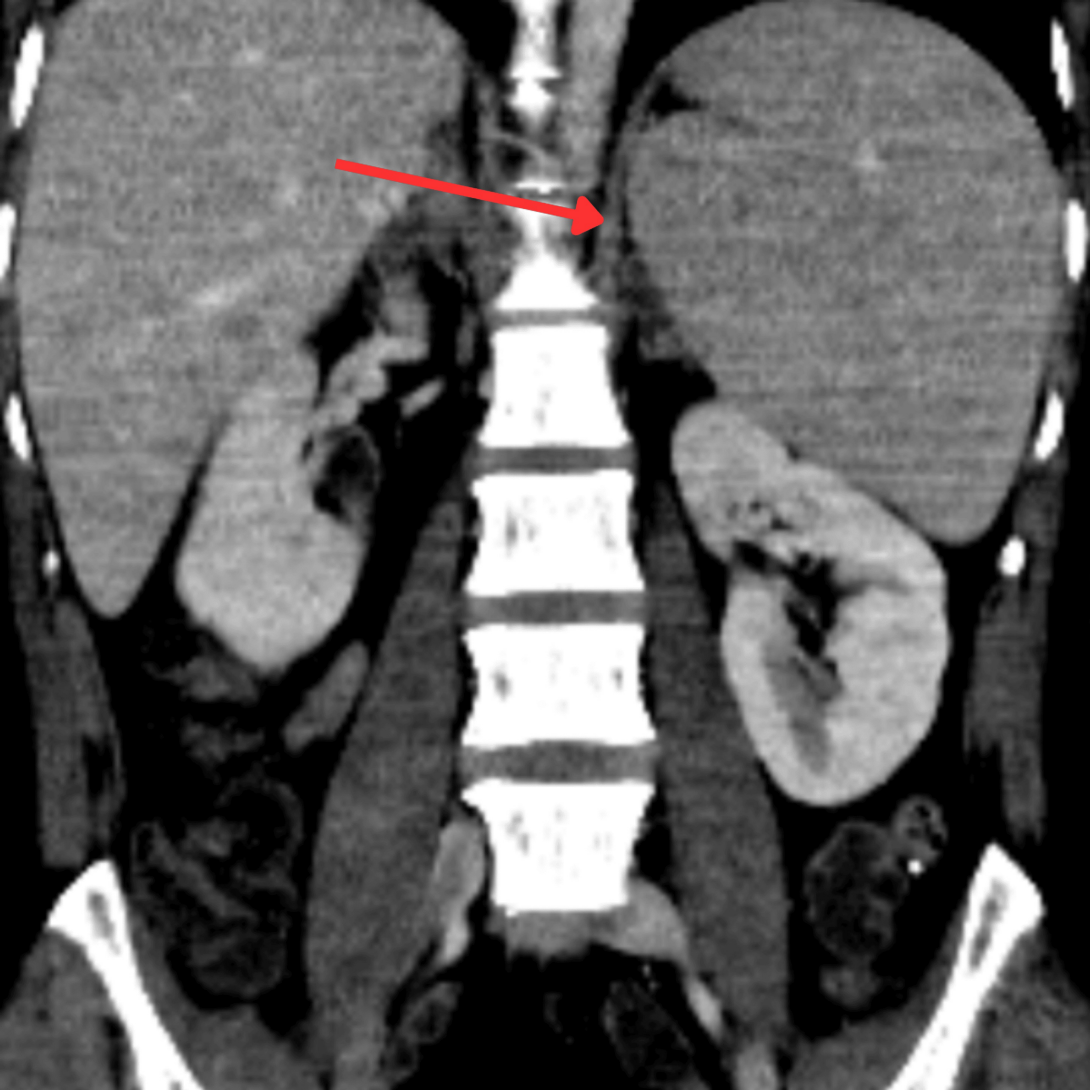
CT abdomen showing splenomegaly (red arrow), supporting disseminated tuberculosis. No focal splenic lesion is seen.
